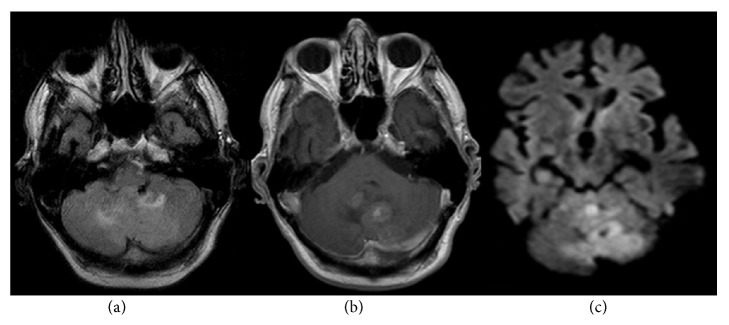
Transversal 1.5 Tesla FLAIR images (a) of a left cerebellar and vermal medulloblastoma show poor tumor delineation and inhomogeneous hyperintensity. Medulloblastomas normally appear hypointense relative to gray matter on T1w images with minimal to patchy contrast enhancement (b) and moderate restriction on diffusion weighted images (c).
